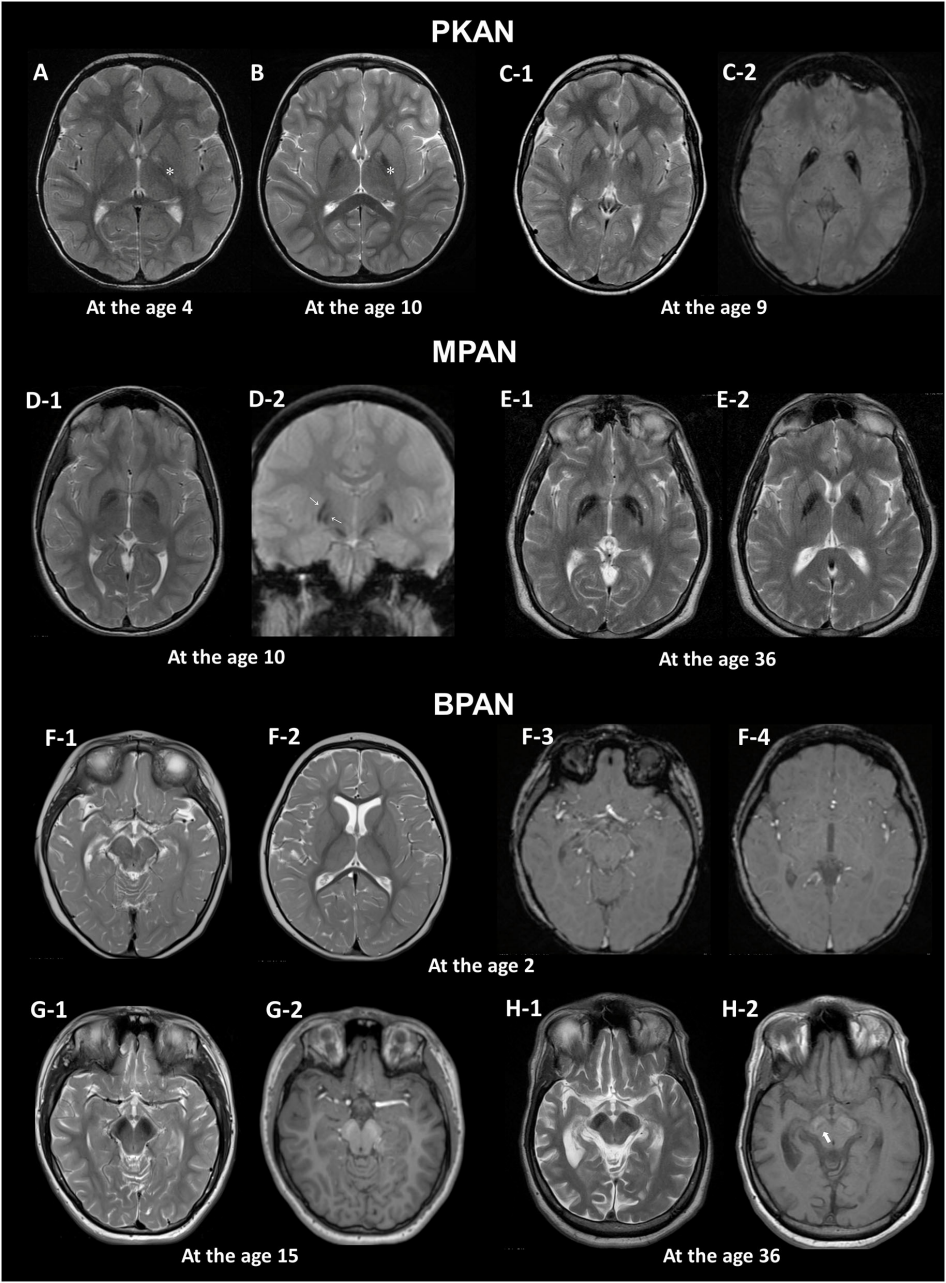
MRI hallmarks of PKAN (A–C), MPAN (D,E), and BPAN (F–H). In a PKAN patient with serial MRIs, the surrounding T2 hypointensity decreases in signal intensity with age (A,B, asterix). The PKAN-specific eye-of-the-tiger sign at the level of the anterior commissure (C-1) is better defined by the region-specific pattern of iron deposition on susceptibility-weighted image (C-2). In a younger patient with MPAN, T2- and T2*-weighted images shows preservation of isointense signal in the middle of inner and outer layers of iron accumulation (D-1, D-2, thin arrows). The typical streaking of the medial medullary lamina on T2-weighted image becomes more pronounced as signal intensity in the GP decreases, which is typically visible at the level of the anterior commissure (D-1, E-1). Brain MRI scans of three BPAN patients at different ages show age-related MRI changes. Brain MRI of a patient with BPAN at the age of 2 shows no abnormality on T2-weighted (F-1, F-2) and susceptibility-weighted (F-3, F-4) images. The substantia nigra is seen as hypointense on T2-weighted image (G-1, H-1) and hyperintense on T1-weighted image, whereas the central hypointense band is not demonstrated (G-2). T1-weighted image demonstrates hyperintensity of the substantia nigra and cerebral peduncle surrounding a central linear band of hypointensity, also known as the halo sign (H-2, thick arrow).
